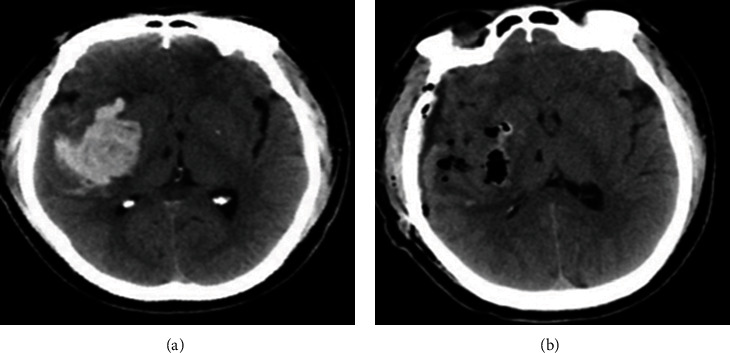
A 54-year-old male patient with hypertensive intracerebral hemorrhage in the posterior basal ganglia region: (a) preoperative CT; (b) postoperative CT.
